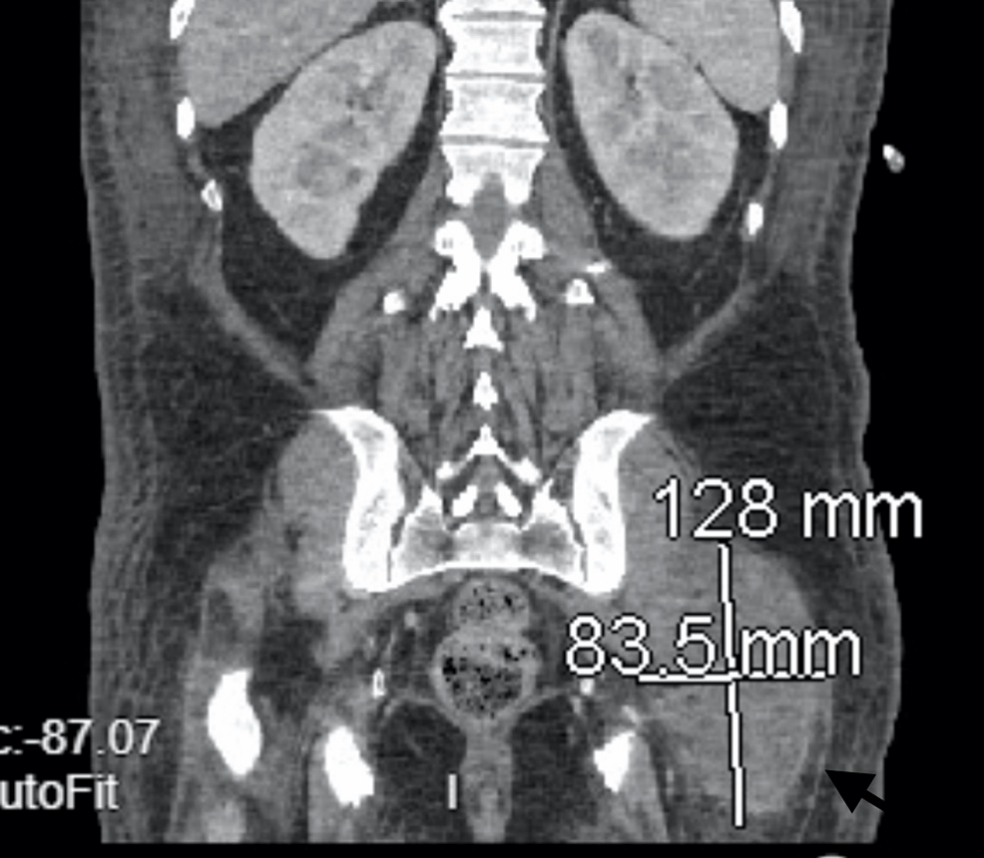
CT scan with coronal view showing new fluid collection with gas in the left gluteal region
